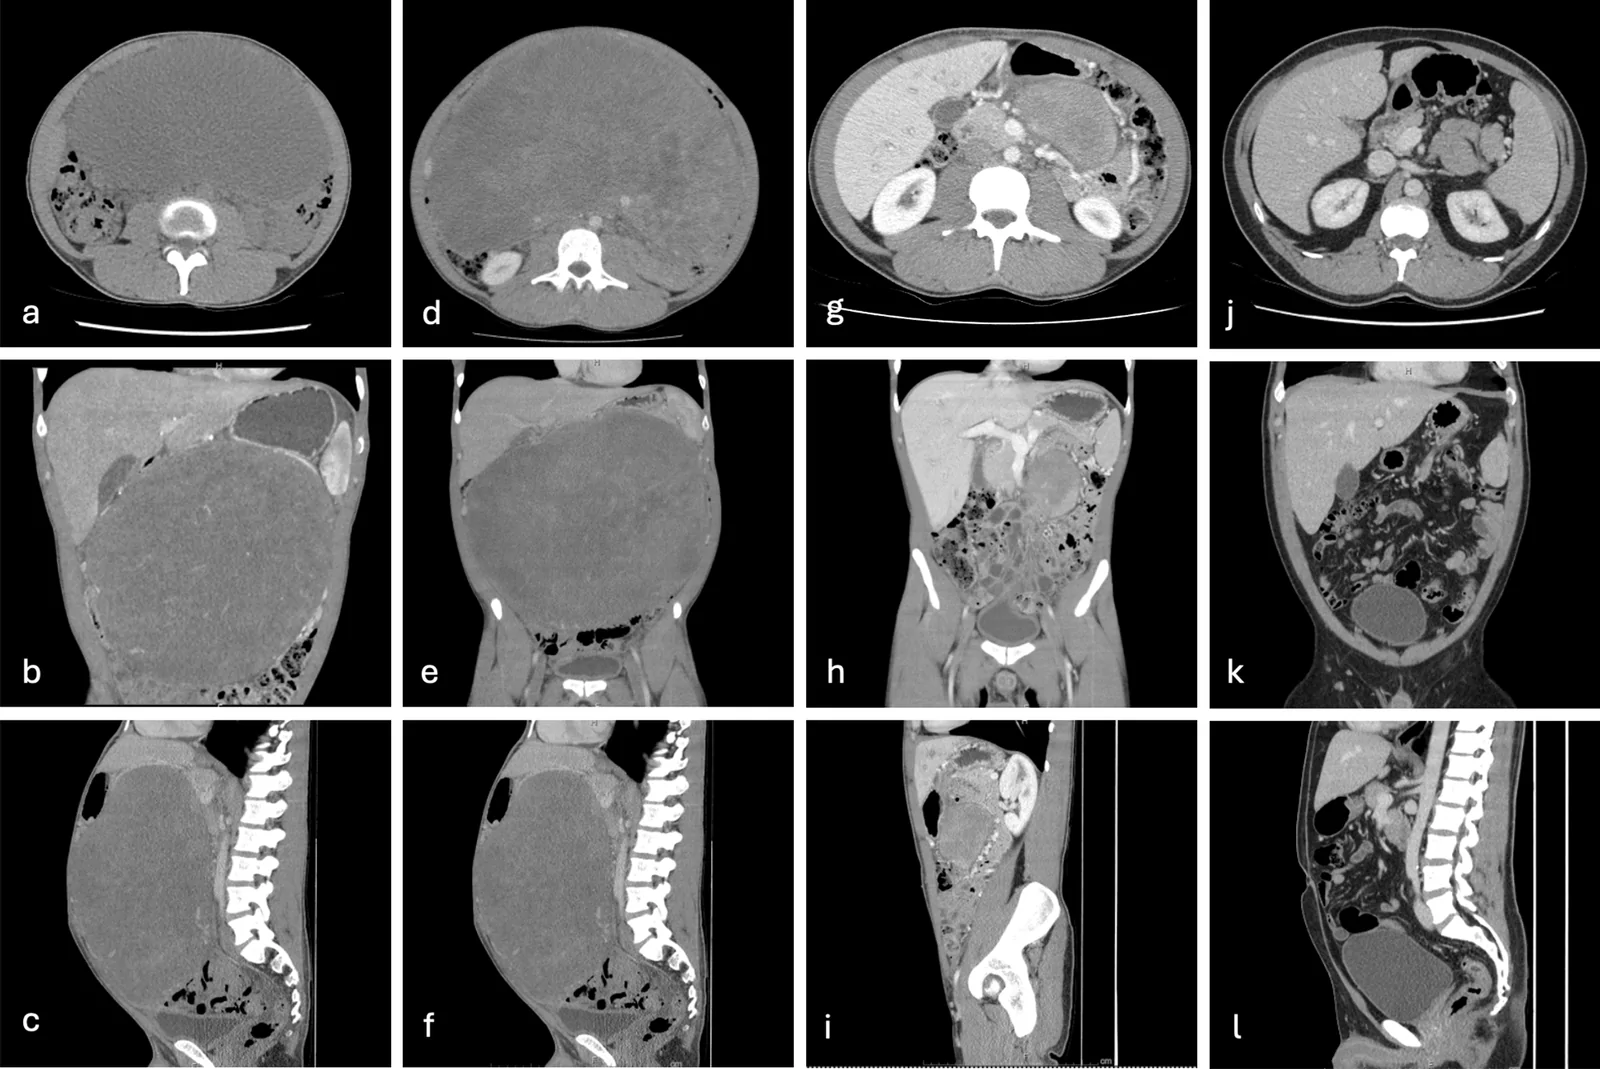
Computed tomography images of mesenteric desmoid tumour during course of treatment. Respective transverse, coronal, sagittal views at diagnosis (a–c), week 25 after combination tamoxifen and suldinac therapy (d–f), week 58 while on second-line imatinib (g–i), and 10.5 years (j–l)
